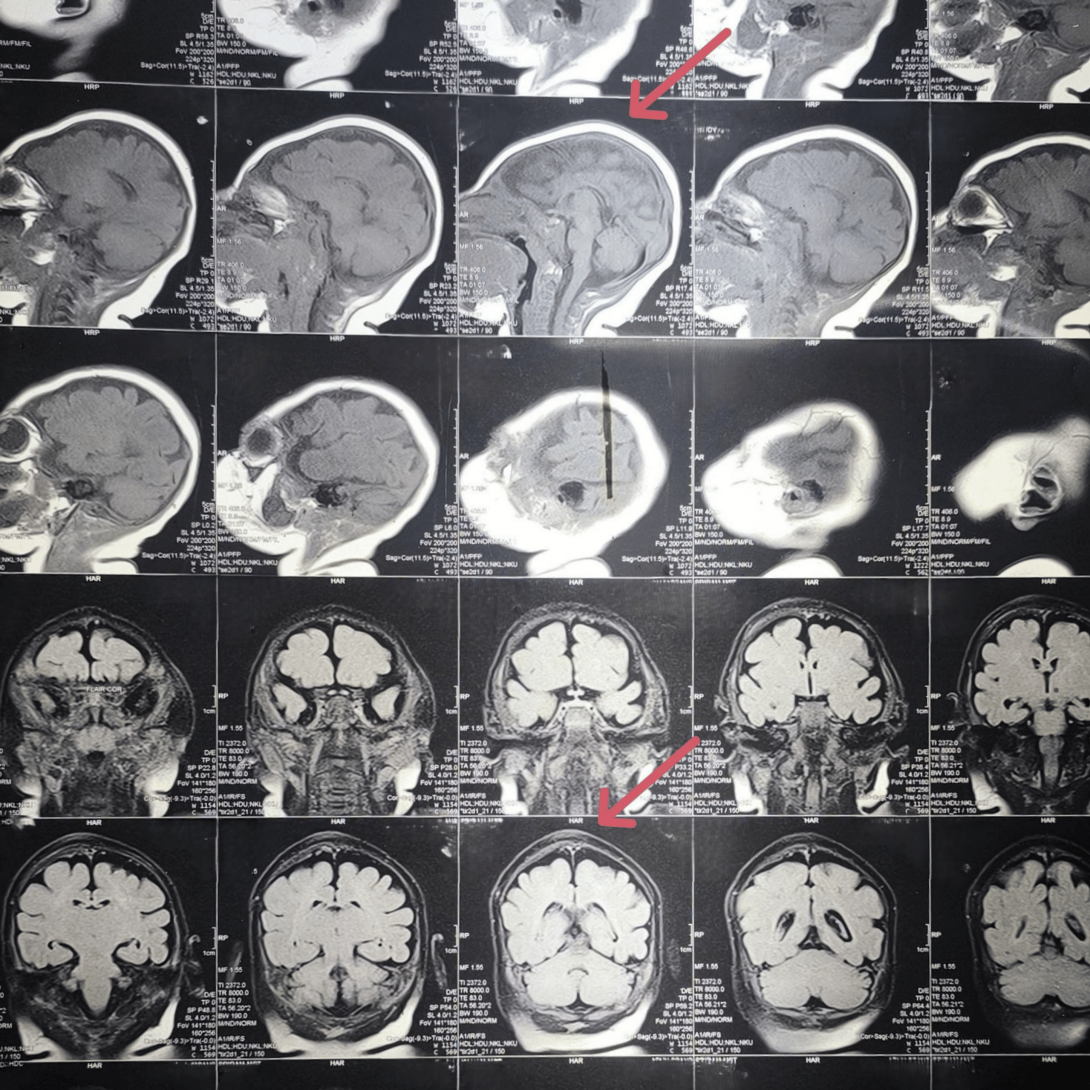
MRI of the brain showing findings suggestive of microcephaly, premature fusion of cranial sutures, and mild diffuse cerebral atrophy
